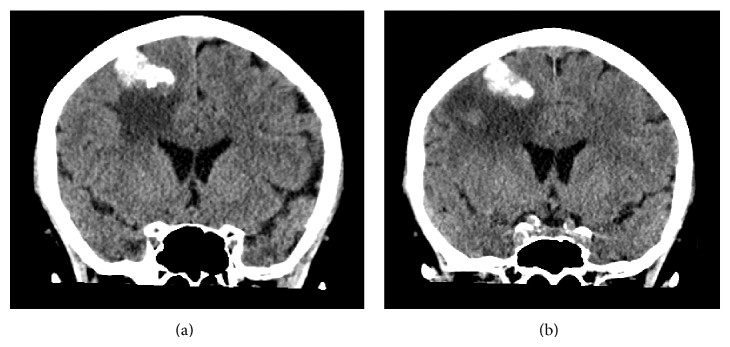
(a) 2014 precontrast CT, coronal view. (b) 2014 postcontrast CT, coronal view.
